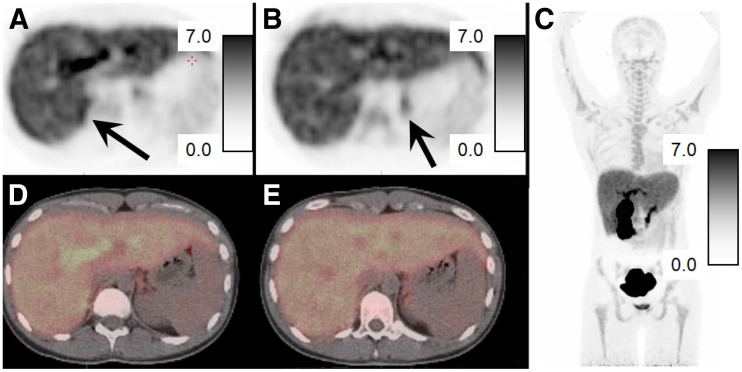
PET/CT images of ^18^F-FNP-59 in a 21-y-old woman without adrenal pathology and pretreated with cosyntropin given before 222 MBq (6 mCi) of ^18^F-FNP-59. (A and B) Axial PET images obtained at 3 h after injection of in upper abdomen, with adrenal glands (black arrows) identified on right (A) and left (B). Scale bars are 0–7 SUV. Adrenal-to-liver ratio at approximately 1.3:1 on right and 1:1.1 on left at 3 h. (C–E) Fused PET/CT images of adrenal glands are also shown (D and E) along with maximum-intensity-pixel image (C) that demonstrates expected gallbladder/biliary/bowel uptake given bile secretion.
